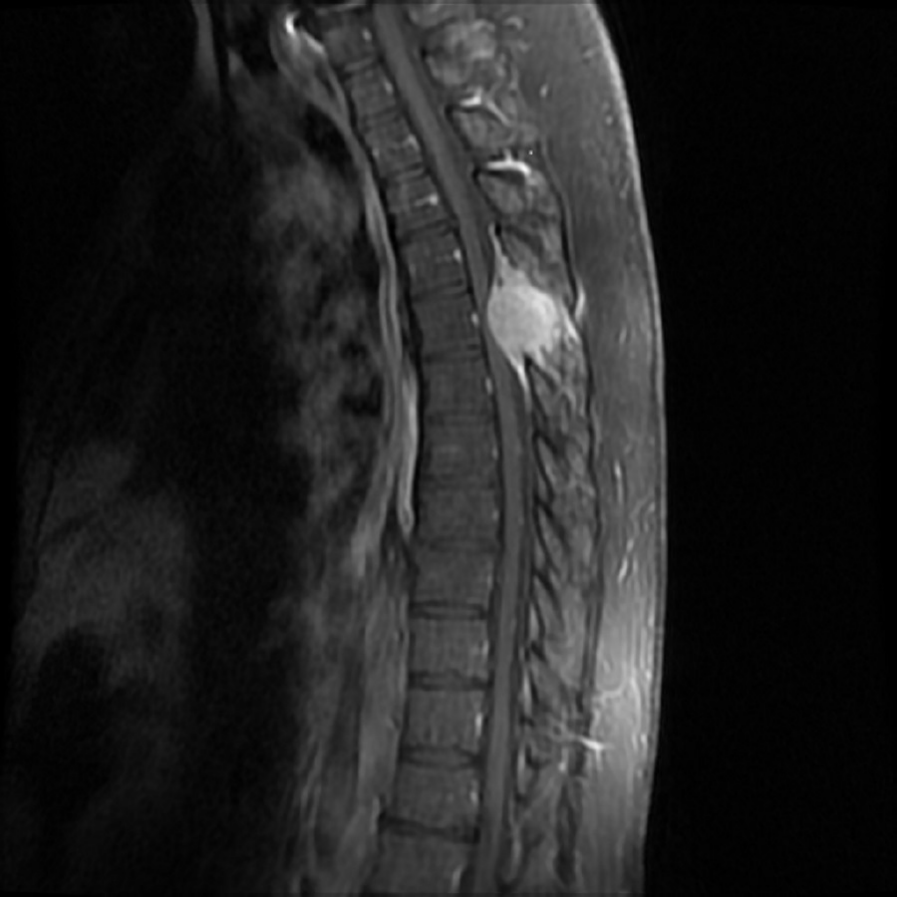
Preoperative Short TI Inversion Recovery (STIR) sagittal images. MRI of the thoracic spine STIR sagittal images revealed an extradural midline mass centered in the posterior elements of T5. The mass was extending into the spinal canal, with severe thoracic cord compression.
